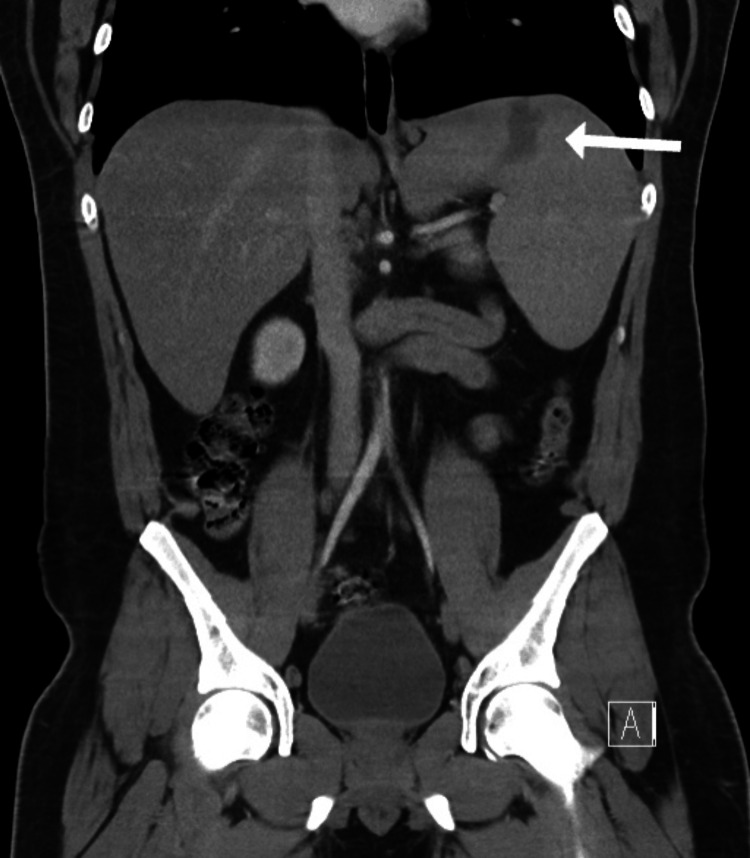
Coronal plane CT scan of abdomen showing splenomegaly and splenic infarction (white arrow)
